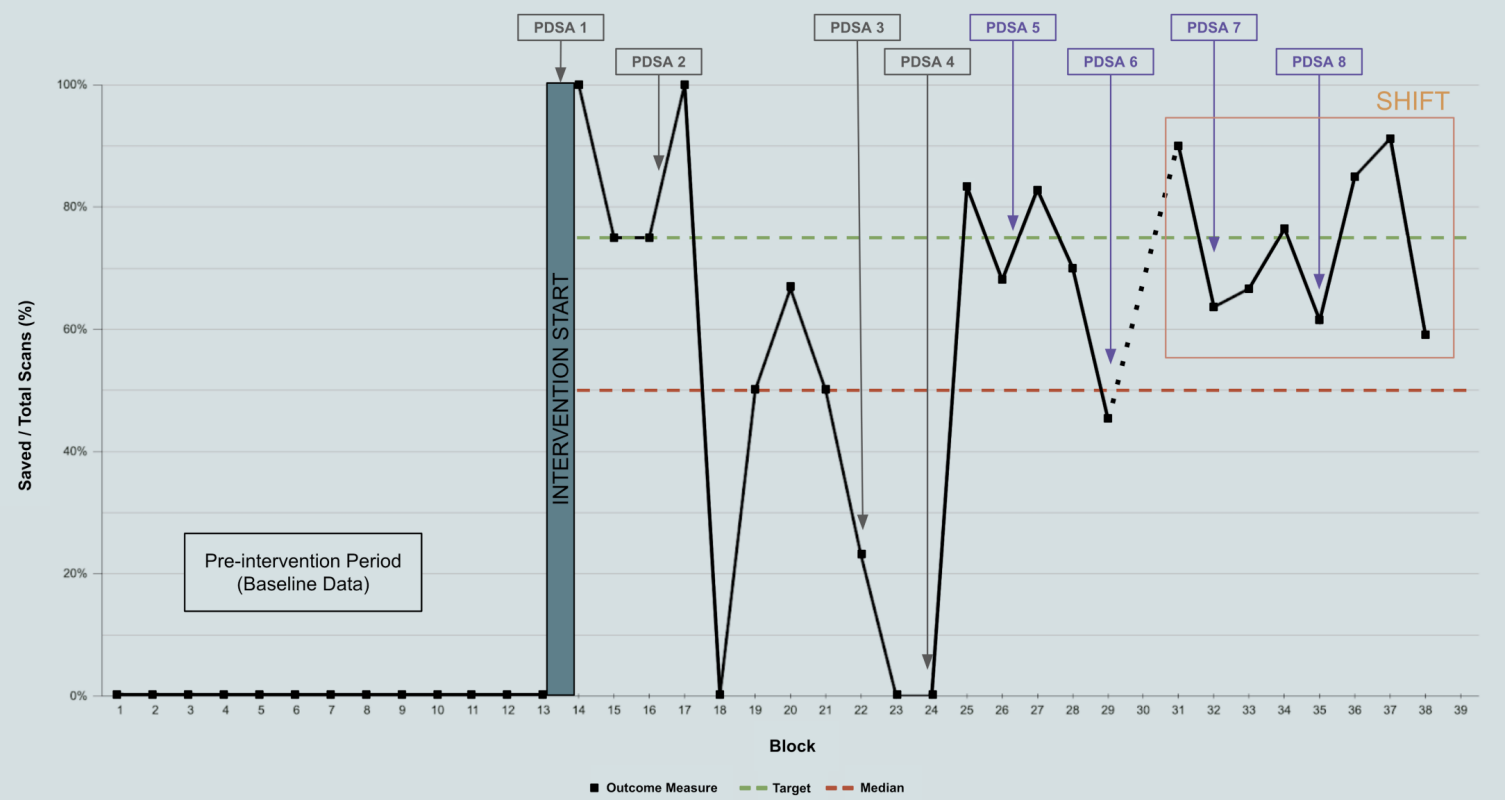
Run chart for the study period. Approximate implementation times of change ideas and Plan-Do-Study-Act (PDSA) cycles are shown. A shift in a positive direction is indicated by the orange box. The dotted line indicates a period with no ultrasound use.
